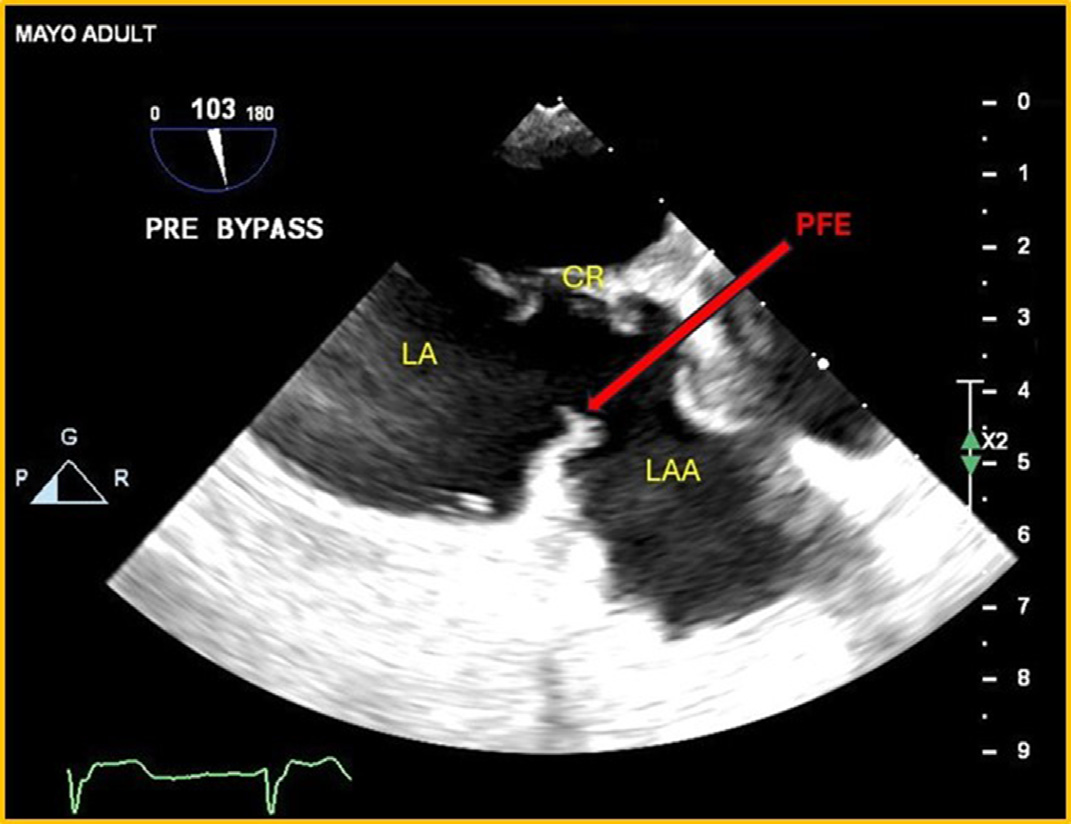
Transesophageal Echo Intraoperative TEE, 2-D mode-focus on LAA and coumadin ridge, dilated LA with a large mobile filamentous mass attached to the orifice of LAA. Abbreviations as in Figures 1 and 2.
